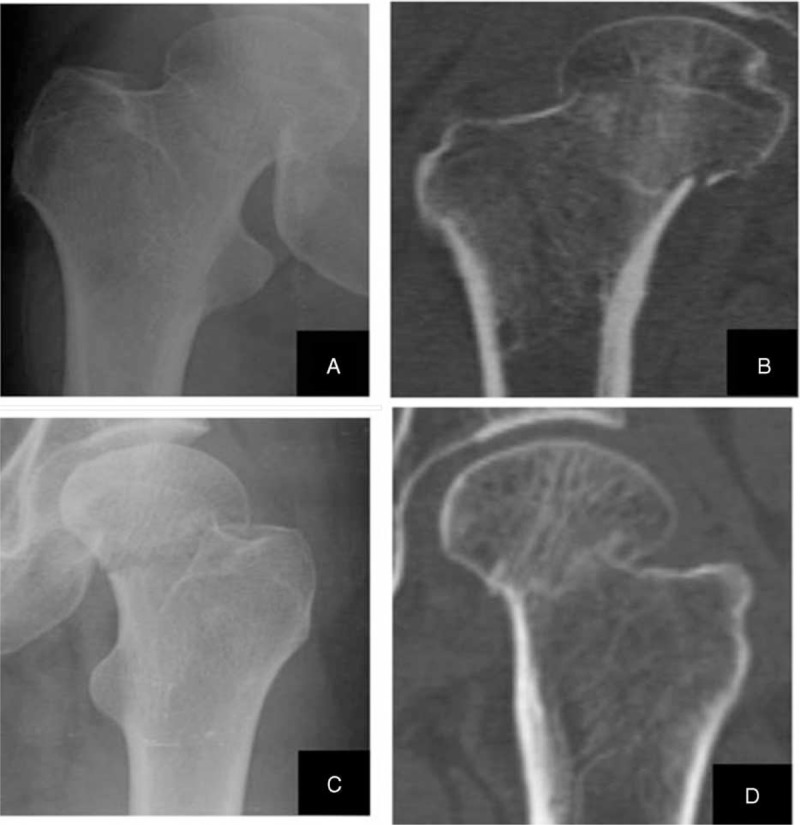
(A) Antero-posterior radiograph of a right hip showing an incomplete femoral neck fracture. (B) Coronal scanning of computed tomography showing a complete fracture. (C) Antero-posterior radiograph of a left hip showing a Garden stage I femoral neck fracture. (D) Coronal scanning of computed tomography shows an impacted femoral neck fracture.
